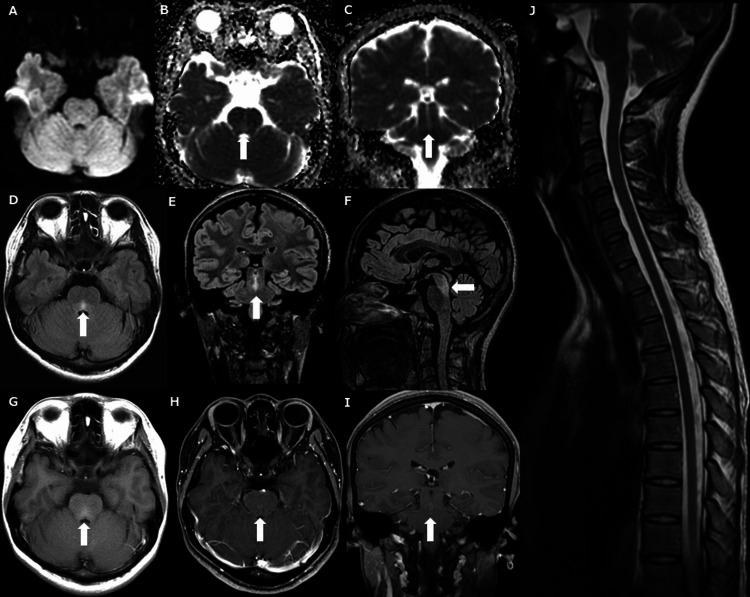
MRI at admission on day 61. Diffusion-weighted imaging (DWI) revealed no lesions (A). Apparent diffusion coefficient (ADC) map indicates a high-intensity lesion extending from the midbrain to the dorsal median pons (arrow) (B and C). Fluid-attenuated inversion recovery (FLAIR) imaging demonstrates a high-intensity lesion extending from the midbrain to the dorsal median pons (arrow) (D, E, and F). T1-weighted images display a high-intensity lesion extending from the midbrain to the dorsal median pons (arrow) (G). Contrast-enhanced T1-weighted images display focal enhancement (arrow) (H and I). Spinal cord MRI reveals no lesions (J). The lesion location can be anatomically explained as corresponding to the patient's diplopia. The presence of a hyperintense lesion on FLAIR with focal enhancement, in conjunction with the absence of signal change on DWI but high signal on ADC map, suggests an inflammatory etiology (brainstem encephalitis) based on imaging findings.
